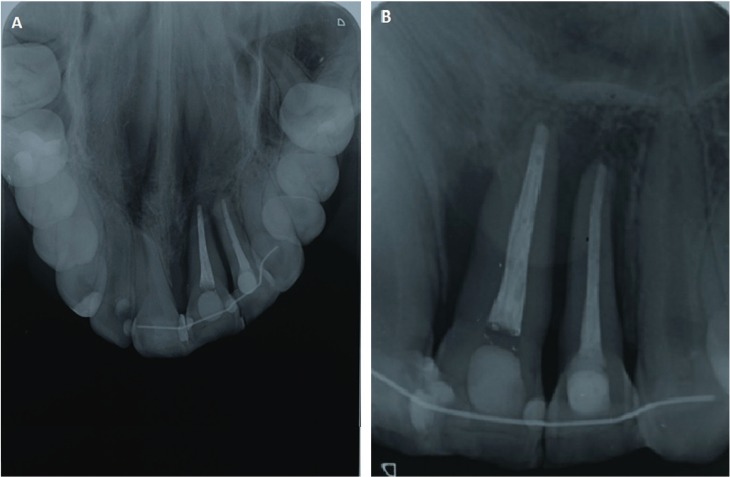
A) Occlusal Radiograph. B) Periapical radiograph with advanced vertical alveolar bone loss.
